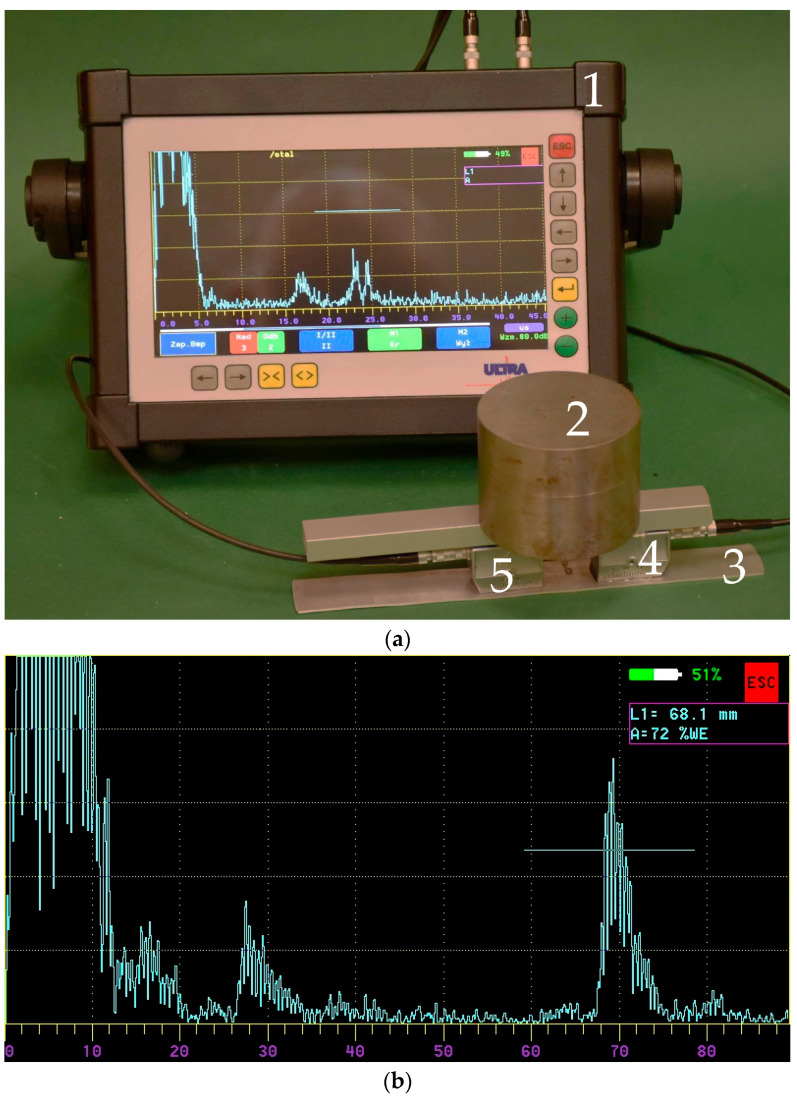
Test using classic surface wave transducers; (a) view of the test station 1—ultrasonic flaw detector, 2—load, 3—test sample, 4—transmitter head, 5—receiver head; (b) view of the screen with the obtained signal.
